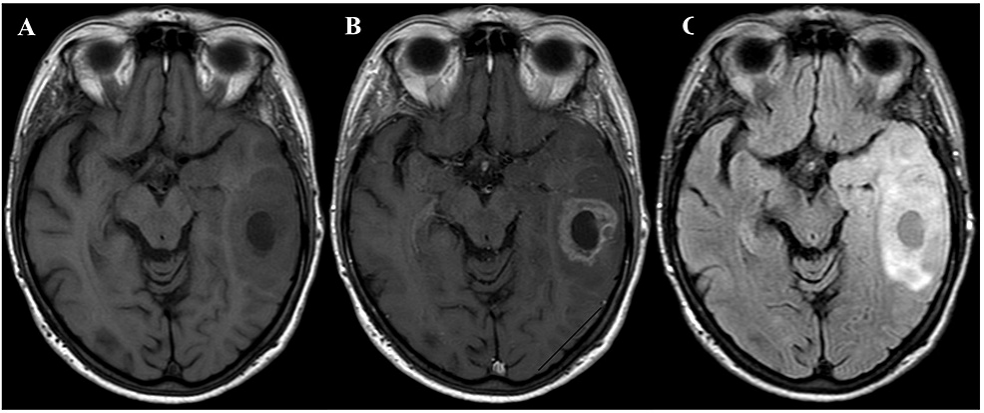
Preoperative MRI of the brain. Axial images of the brain showing a left temporal lobe glioma in close proximity to Wernicke’s area and the arcuate fasciculus, with significant vasogenic edema, and a 3-4 mm left to right midline shift. (A) T1 without contrast, (B) T1 with contrast, and (C) fluid-attenuated inversion recovery (FLAIR).
